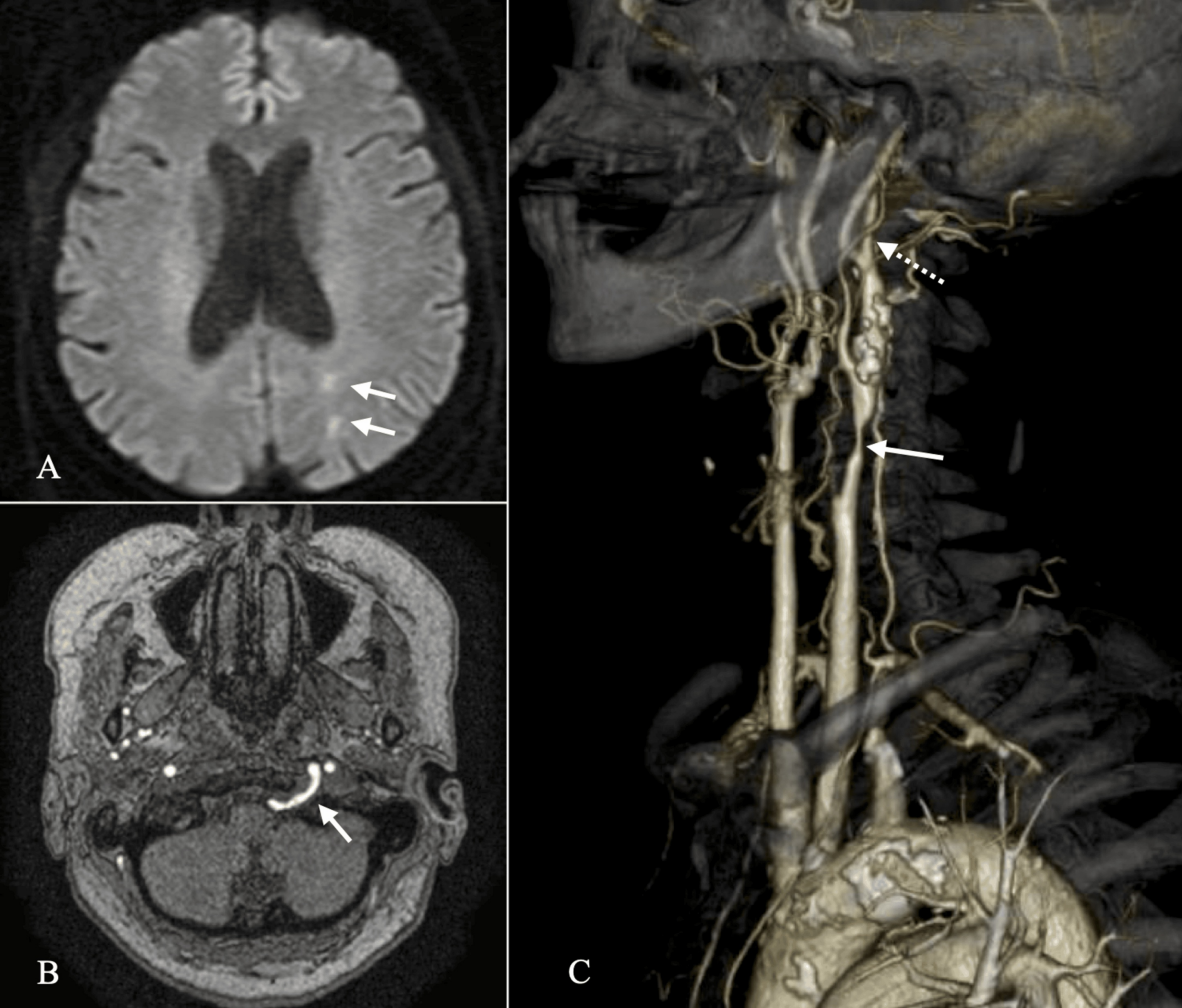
Preoperative MRI and CT angiography. (A) DWI showing hyperintensity at the left parietooccipital area (white arrows). (B) MR angiography showing PPHA running through the left hypoglossal canal (white arrow). (C) CT angiography revealing CCA stenosis (white arrow). PPHA originates from the ICA at the level of the mandibular angle (white dotted arrow) DWI: diffusion-weighted imaging; PPHA: persistent primitive hypoglossal artery; CCA: common carotid artery
